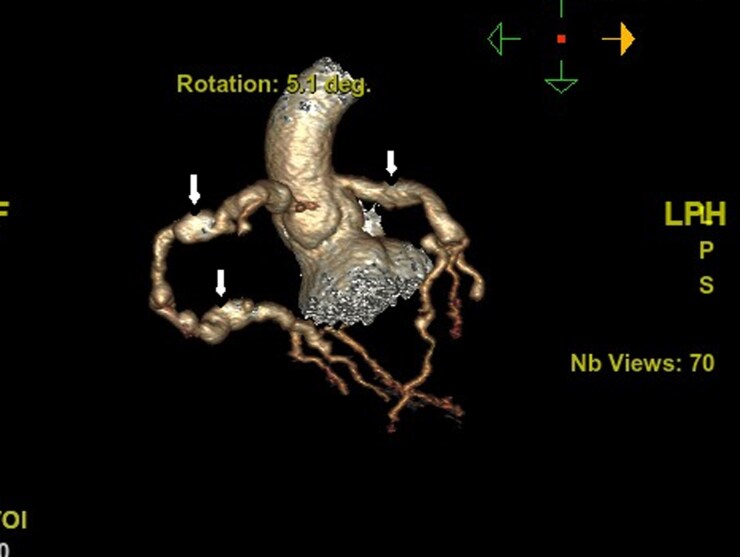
Reconstructed computed tomography image showing aneurysms involving both left anterior descending and right coronary artery. Arrows outline the fusiform and saccular aneurysms.
